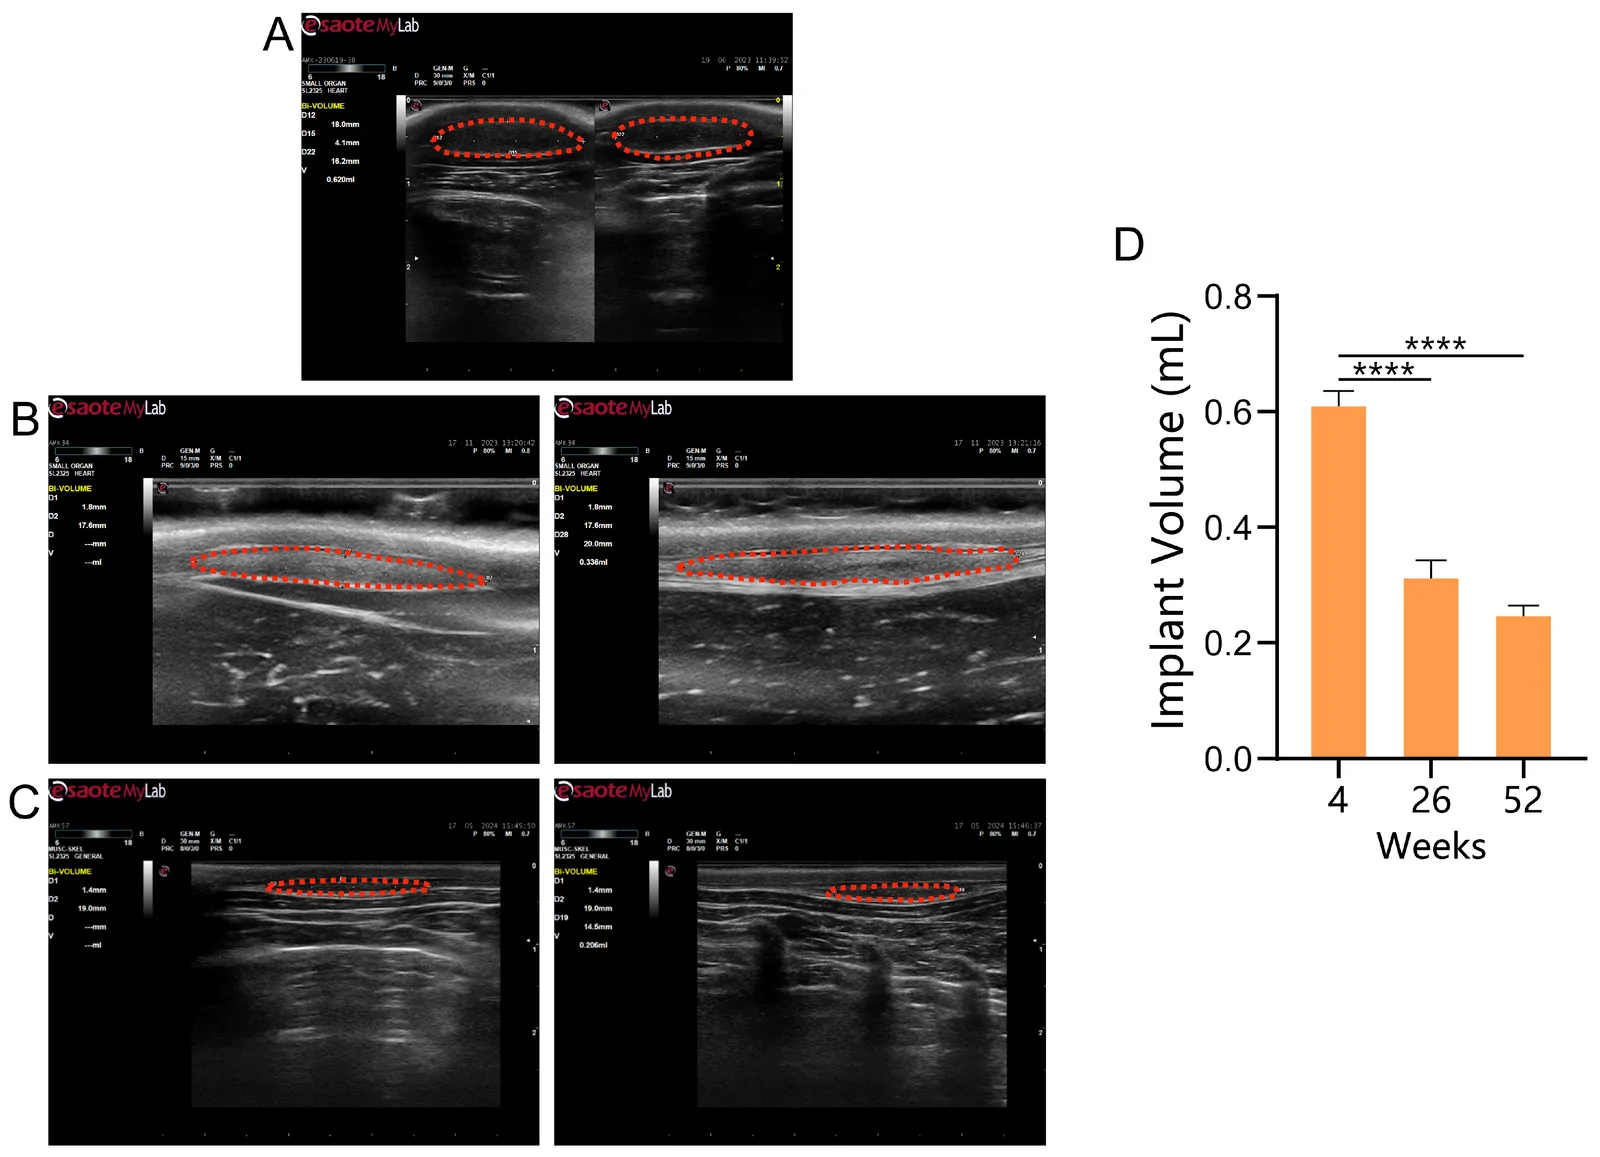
Implant volume of material detected by B-ultrasound. (A) Implant volume at 4 weeks after injection (dotted red line). (B) Implant volume at 26 weeks after injection (dotted red line). (C) Implant volume at 52 weeks after injection (dotted red line). (D) Implant volume statistics at different times after injection.  *p* < 0.0001, n =  6. Data are presented as mean  ±  SEM. SEM, Standard error of the mean.
